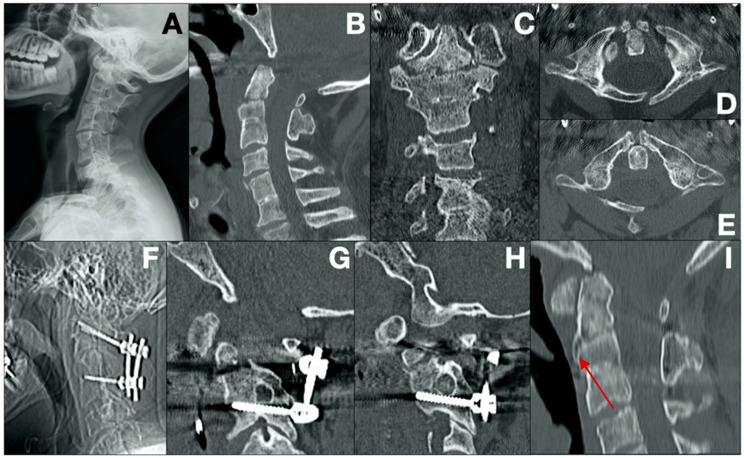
Pre-operative radiographic acquisition (A). Pre-operative sagittal, coronal, and axial CT scans, respectively (B–E). Intra-operative radiographic acquisition (F). 12-month follow-up sagittal CT scans demonstrating osteosynthesis achievement (red arrow) and correct screw alignment in the C3 peduncles (G–I).
